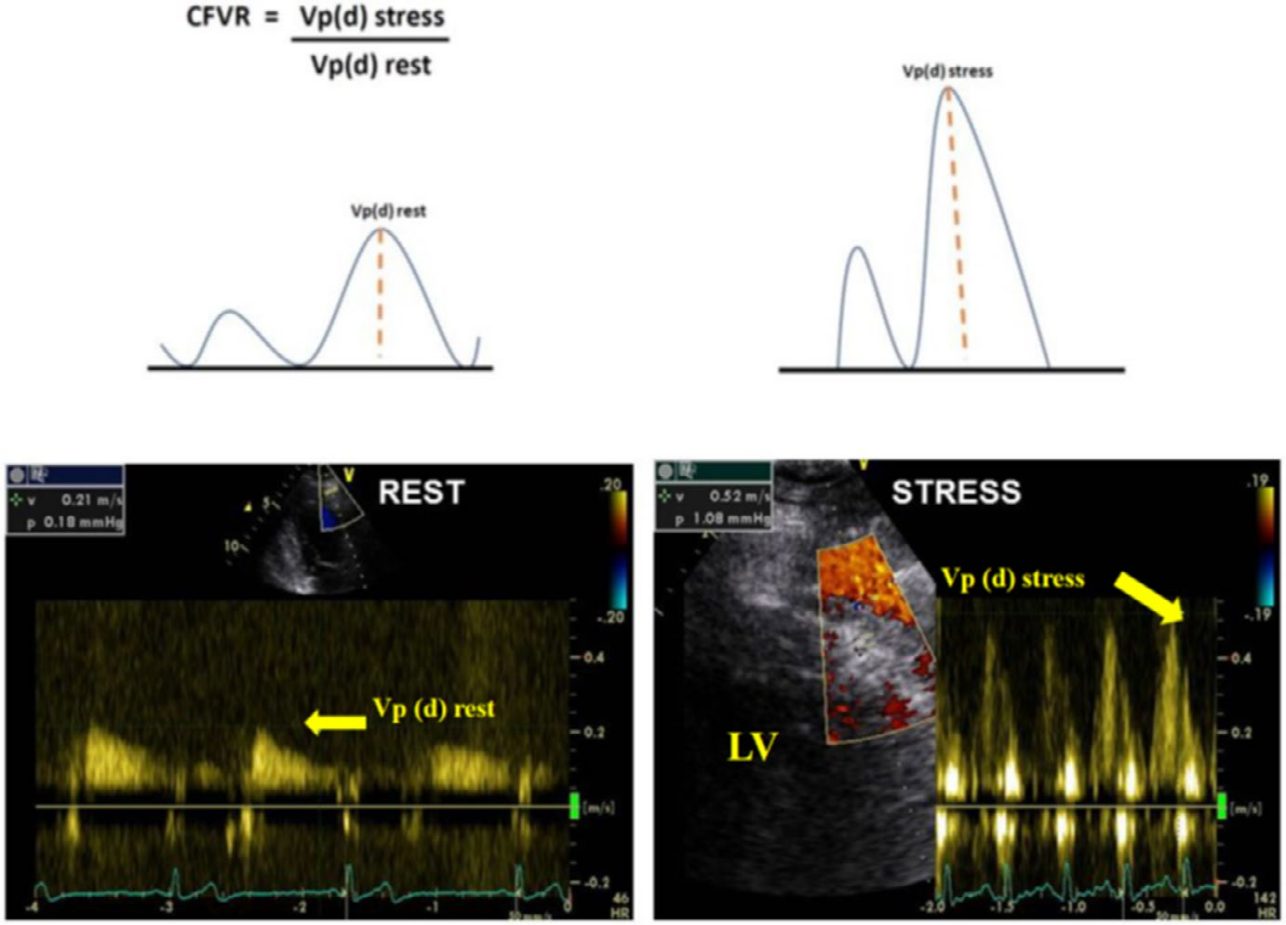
an example of normal coronary flow reserve in a healthy subject. Velocity patterns are registered by pulsed‐wave Doppler. Resting laminar peak diastolic velocity in normal coronary artery is from 0.21 ± 0.08 m/s to 0.28 ± 0.09 m/s, and the velocity will not exceed 1 m/s even in case of its three‐ fourfold increase in the stenosis site. CFR is expressed as the ratio of coronary flow velocity under maximal vasodilatation to coronary flow velocity at rest. In this case, the basal peak diastolic flow velocity is normal, and it increases more than 2 times after dipyridamole, and therefore, CFR is normal. LV = left ventricle; Vp(d) = velocity peak (diastolic); CFVR = coronary flow velocity ratio
